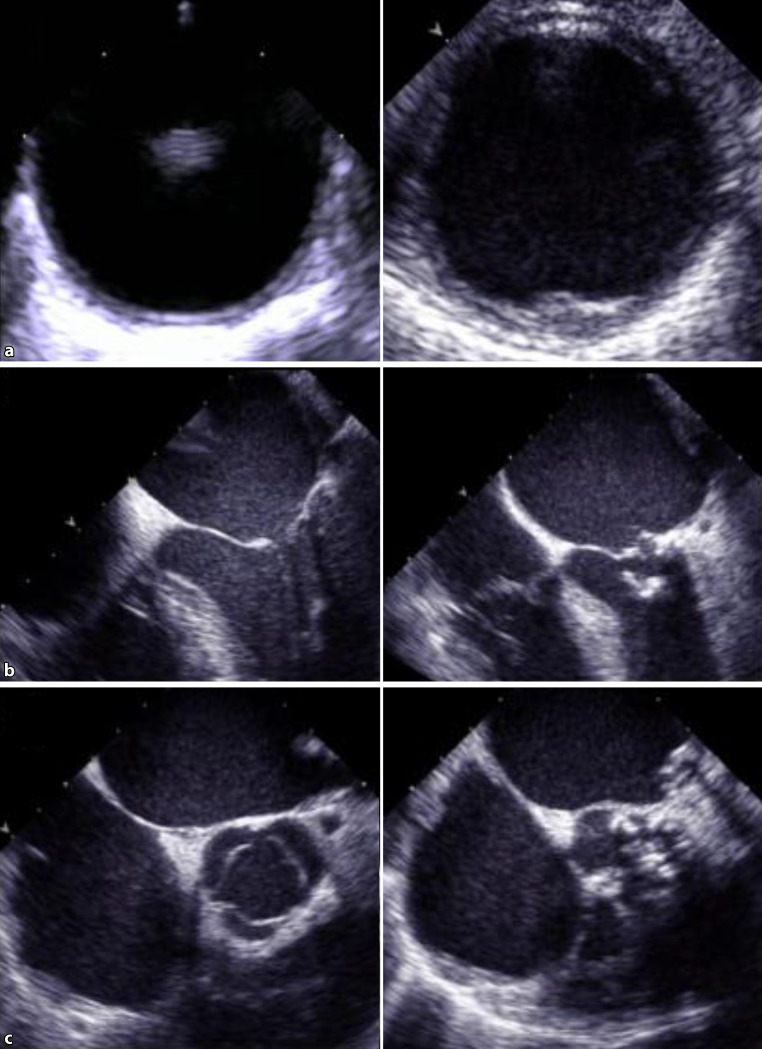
Representative images displaying a transesophageal short axis view of the descending thoracic aorta (panel a) without (*left side*) and with (*right side*) aortic plaque, a transesophageal four-chamber view (panel b) with normal (*left side*) and severely calcified (*right side*) mitral valve, and a transesophageal short axis view of the aortic valve (panel c) with normal (*left side*) and severely calcified (*right side*) morphology
